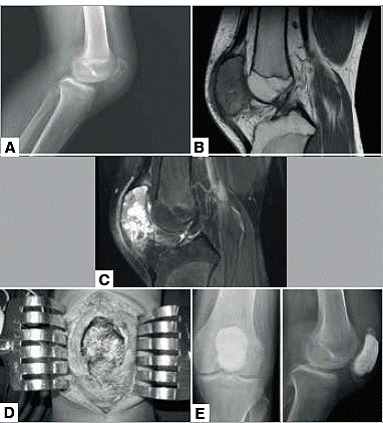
Aneurysmal bone cyst (case 8). (A) Profile x-ray of the knee; (B) Sagittal NMR T1; (C) Sagittal NMR T2; (D) Intraoperative; (E) AP+P/Postoperative x-ray.
